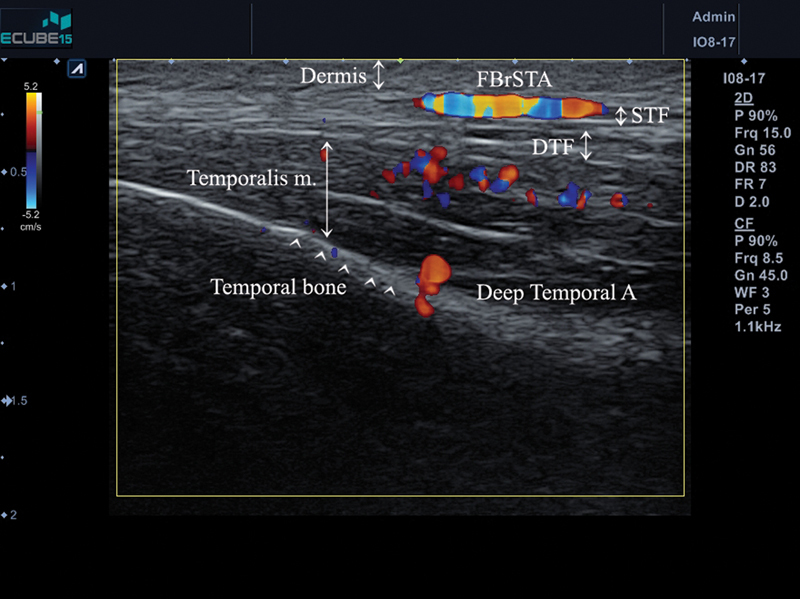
Doppler ultrasound of the temple area. The frontal branch of superficial temporal artery and anterior branch of deep temporal artery are visible.
